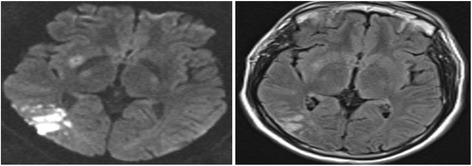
Brain MRI showing right parieto-occipital cortical border zone infarction
